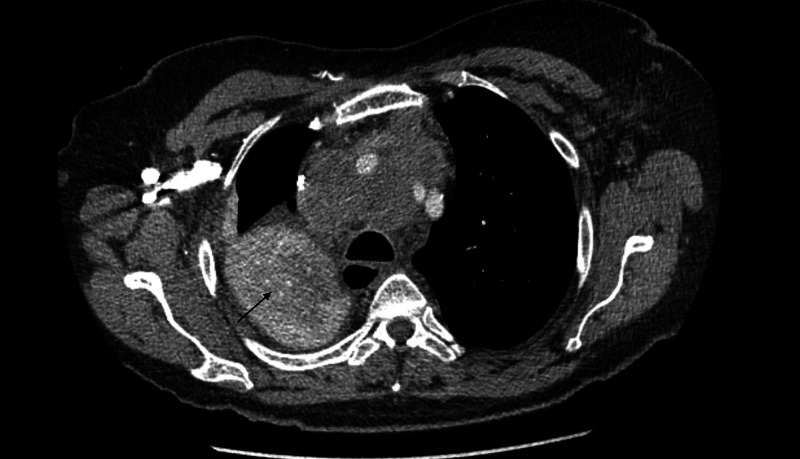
Axial CT scan chest The arrow shows a partially collapsed right upper lobe of the lung due to extrinsic compression of the right upper lobe bronchus from the bulky mediastinal nodes. CT: computed tomography
